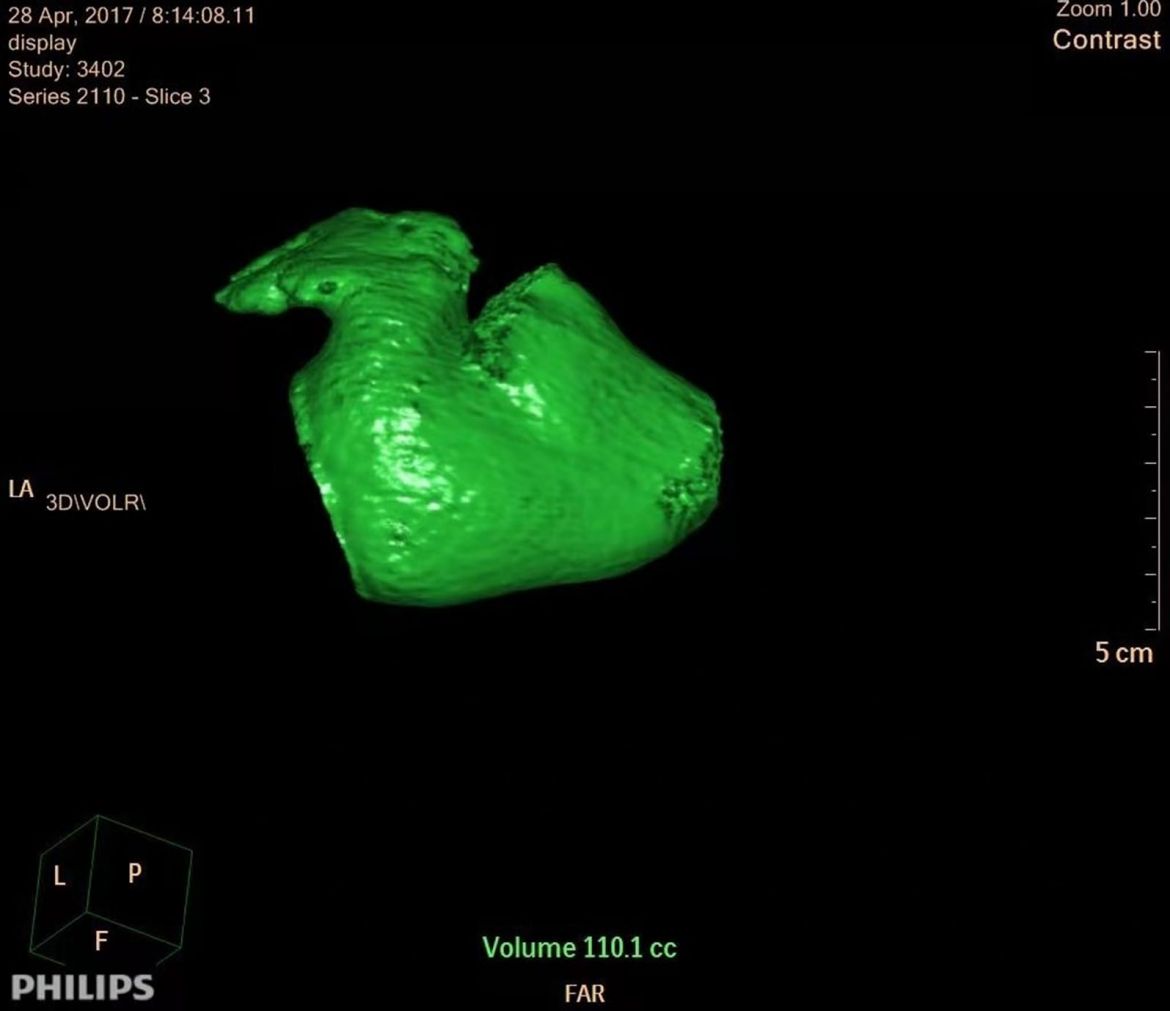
Three-dimensional volume of the left atrium in CT
